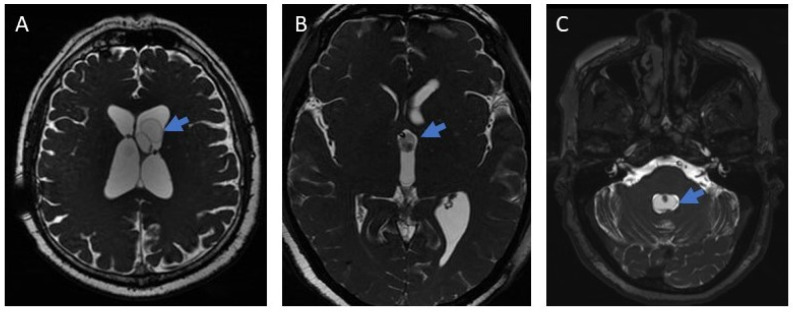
Images of ventricular neurocysticercosis (3D MRI). (A): Lateral ventricle; (B): Third ventricle; (C): Fourth ventricle. The arrows indicate the parasite in each figure.
